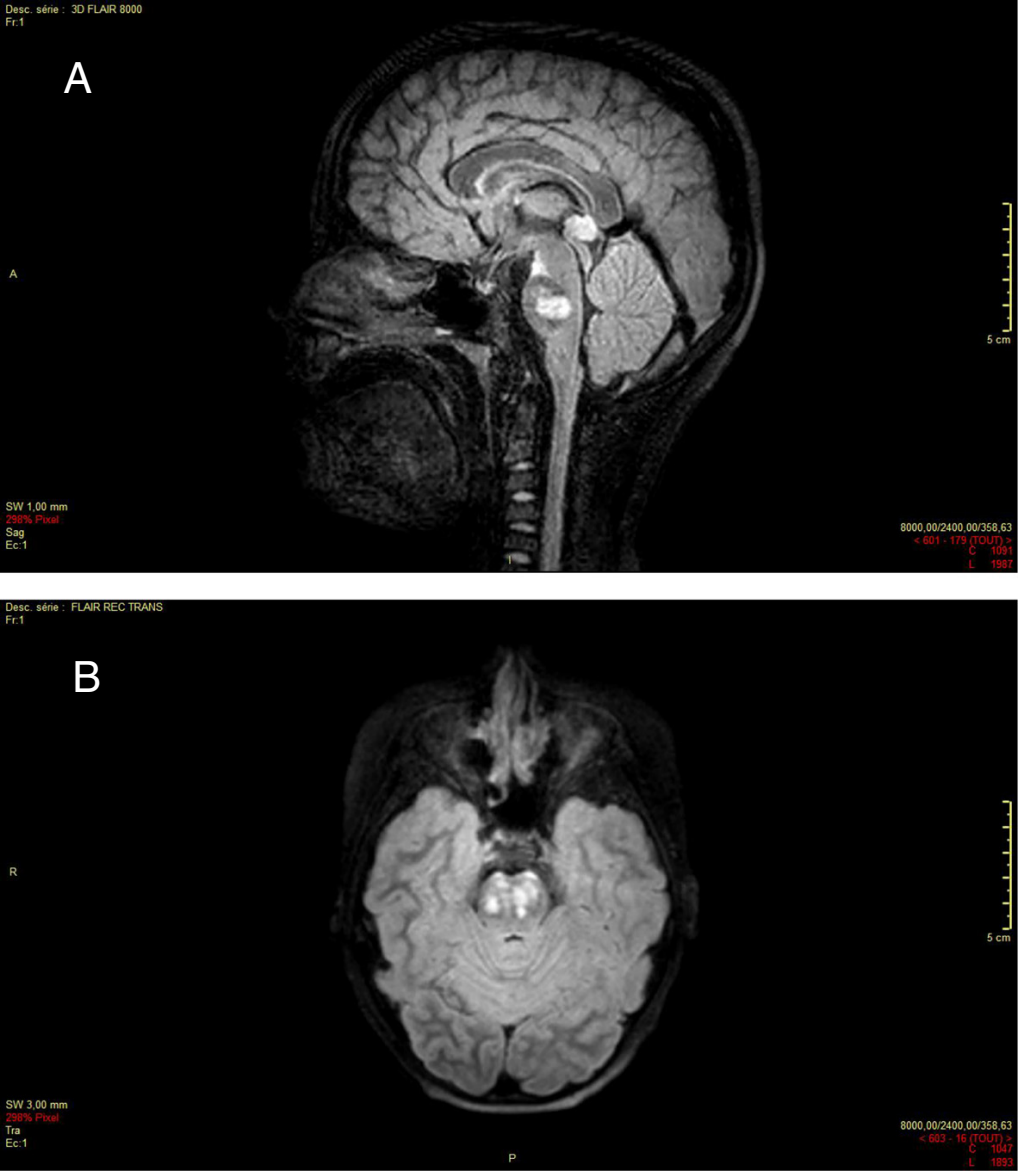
a Bilateral ischemic lesion of the ponto-mesencephalic junction, enhanced on the left side (day 1), 3d FLAIR, sagittal section. b Bilateral ischemic lesion of the ponto-mesencephalic junction, enhanced on the left side (day 1), axial section
